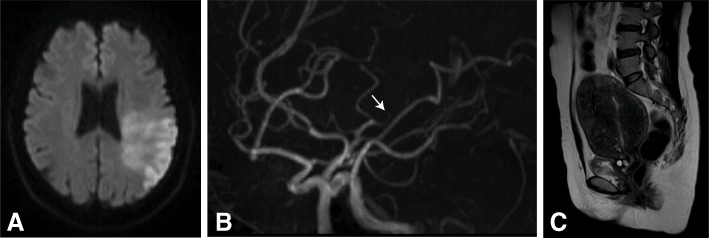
a Diffusion-weighted magnetic resonance imaging (MRI) revealed an infarct in the left middle cerebral artery territory. b Magnetic resonance angiography showed occlusion at left M2 (arrow). c T2-weighted pelvic MRI revealed enlargement of the uterus and obscure junctional zone, suggesting adenomyosis
